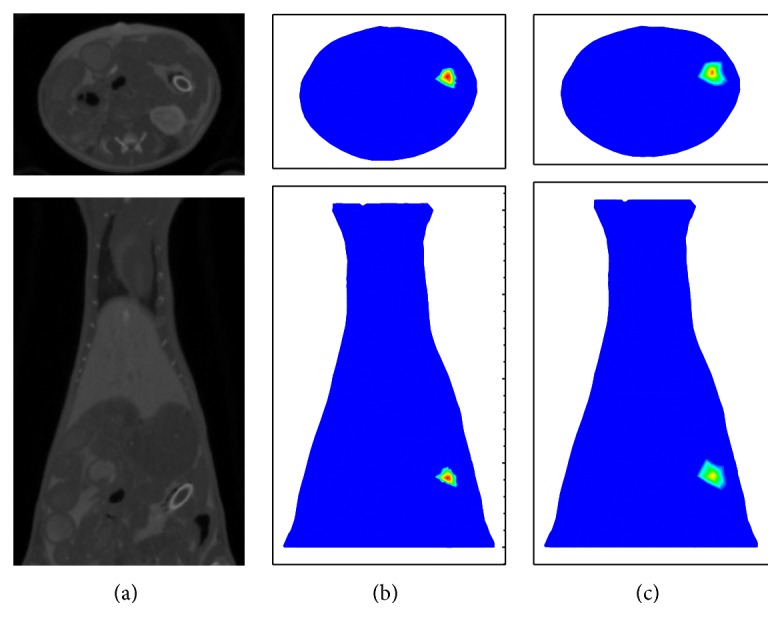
In vivo experiment results. (a) CT slices where the glass tube is the true target. (b) Recovered slices by the SCP-Kaczmarz method. (c) Recovered slices by the Kaczmarz method.
